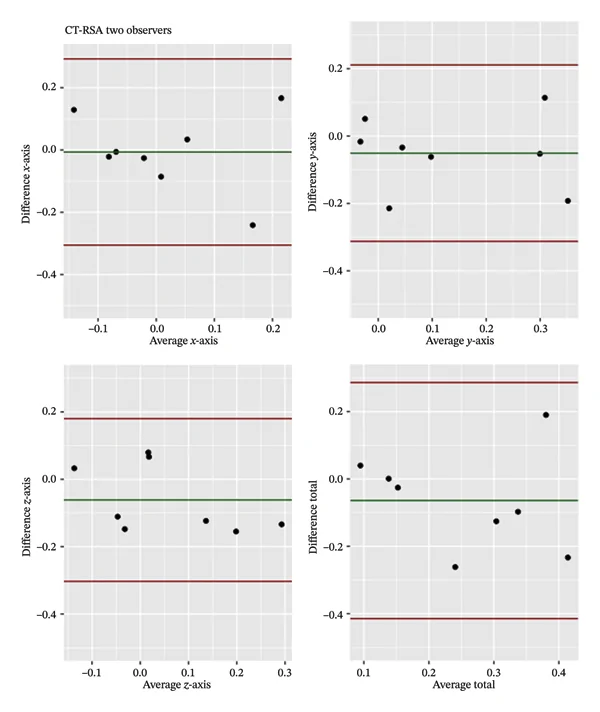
Inter‐rater agreement for wear measurements in *x*, *y*, *z* translations and total translation between two independent observers using CT‐RSA. The green line represents the mean difference and the red lines represent the upper and lower limits of agreement (mean difference ± 2.365 × SD).
